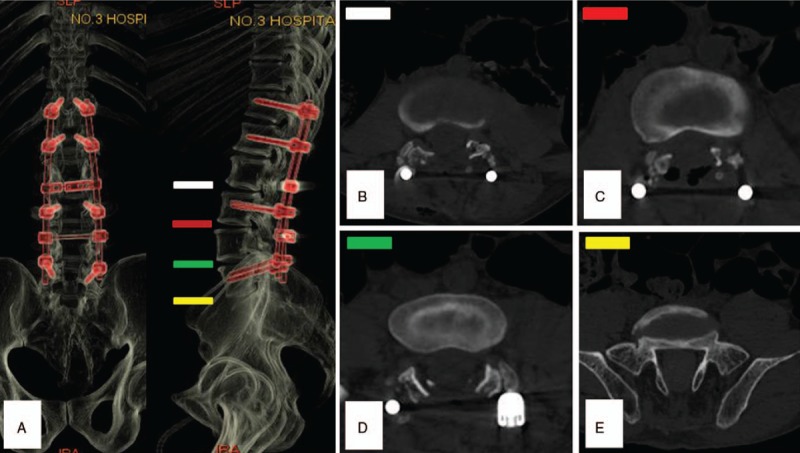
(A) The computed tomography (CT) volume reconstruction post OR. (B–E) Axial CT demonstrated decompression from L3 to L5.
